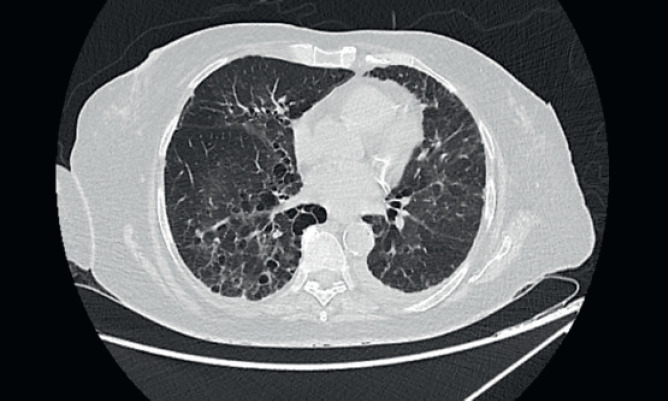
High-resolution CT thorax of the patient prior to initiation of CDK 4/6 inhibitor (Palbociclib).
